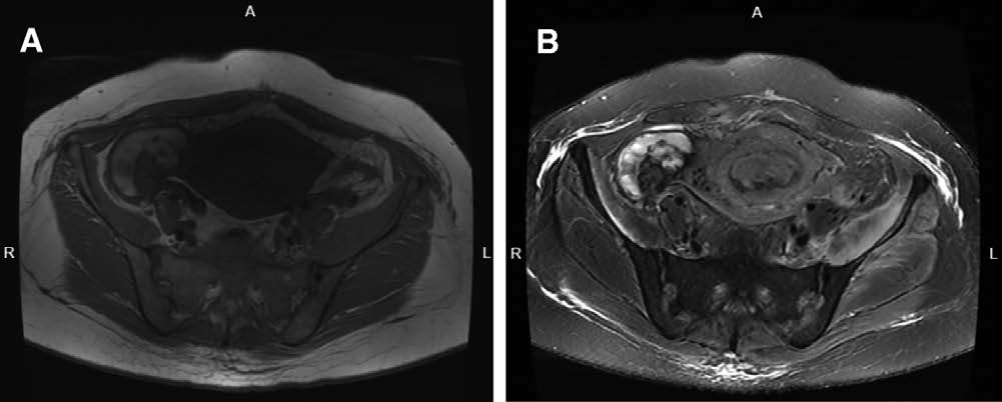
T1- and T2-weighted MRI images of a tortuous strip on the right side of the pelvis. (A) A short T1 signal shadow is observed on the tortuous strip. (B) The same tortuous strip displays a short T2 signal shadow. MRI = magnetic resonance imaging.
